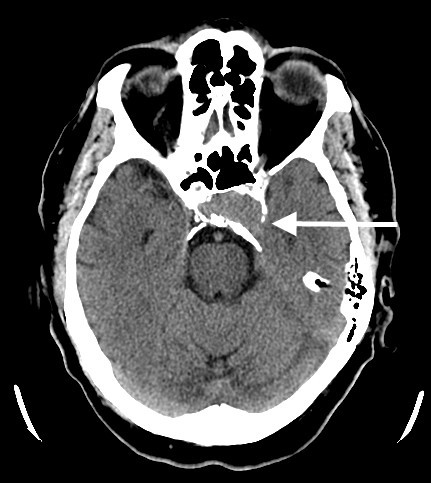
CT without contrast demonstrates a soft tissue attenuation mass filling the sella with adjacent bone remodeling (arrow).
